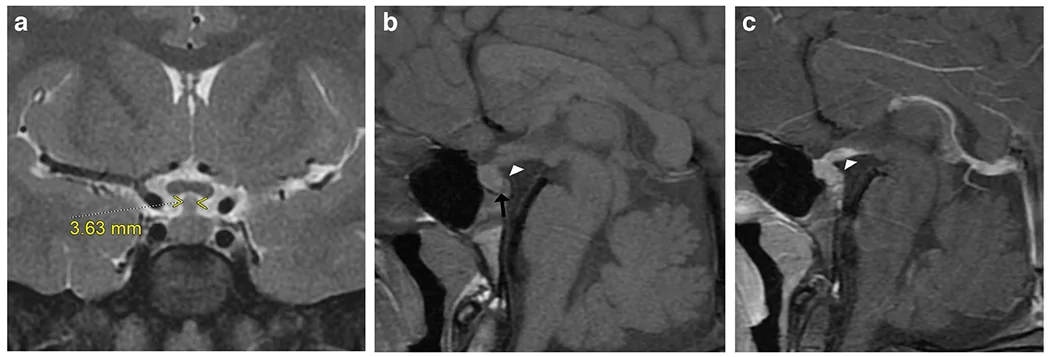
Eight-year-old female with central diabetes insipidus that resolved following therapy for presumptive Langerhans cell histiocytosis. a Coronal T2-weighted sequence through the sella shows abnormally thickened pituitary infundibulum, measuring 3.6 mm. Sagittal (b) pre-contrast and (c) post-contrast T1-weighted images of the sella redemonstrate abnormal infundibular thickening and enhancement to the level of the tuber cinereum (*white arrowheads*). Note also the absence of the posterior pituitary bright spot (*black arrow* in b). Follow-up imaging exams after a chemotherapy course show normalization of the infundibulum and no intrasellar mass (not shown)
